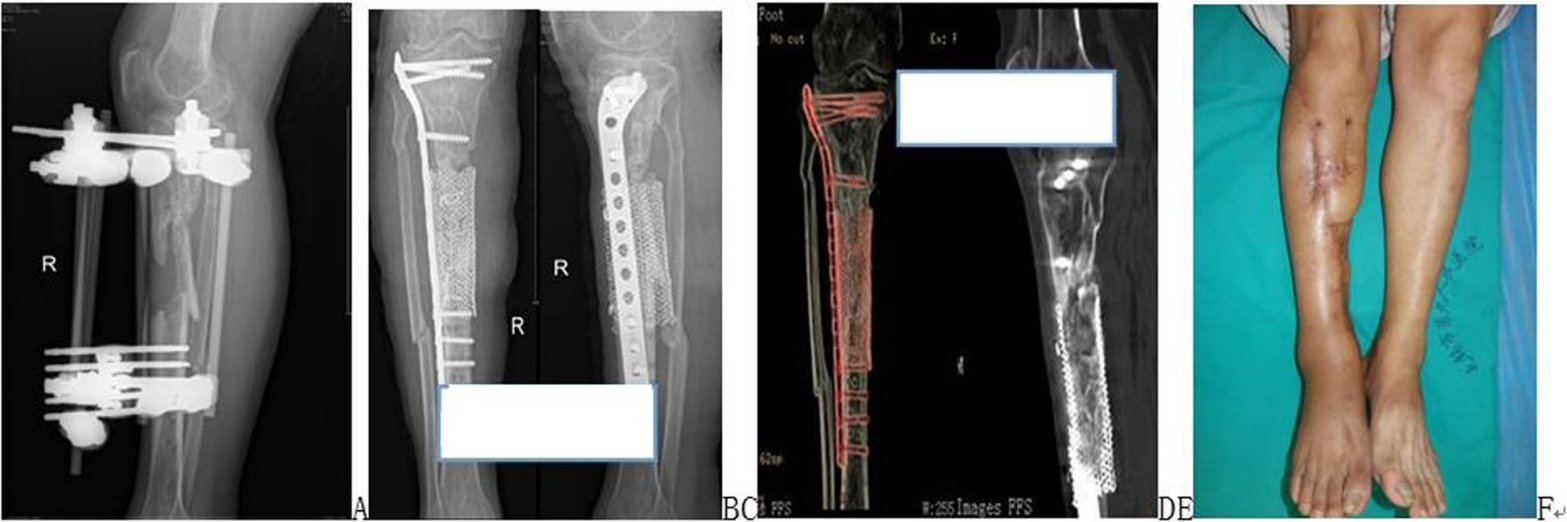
A patient with right tibial fractures combined with bone defects treated with titanium mesh wrapped cancellous bone grafting. a Preoperative X-ray films. b–e Postoperative X-ray films and CT show healing. f Postoperative appearance 1 year postsurgery
